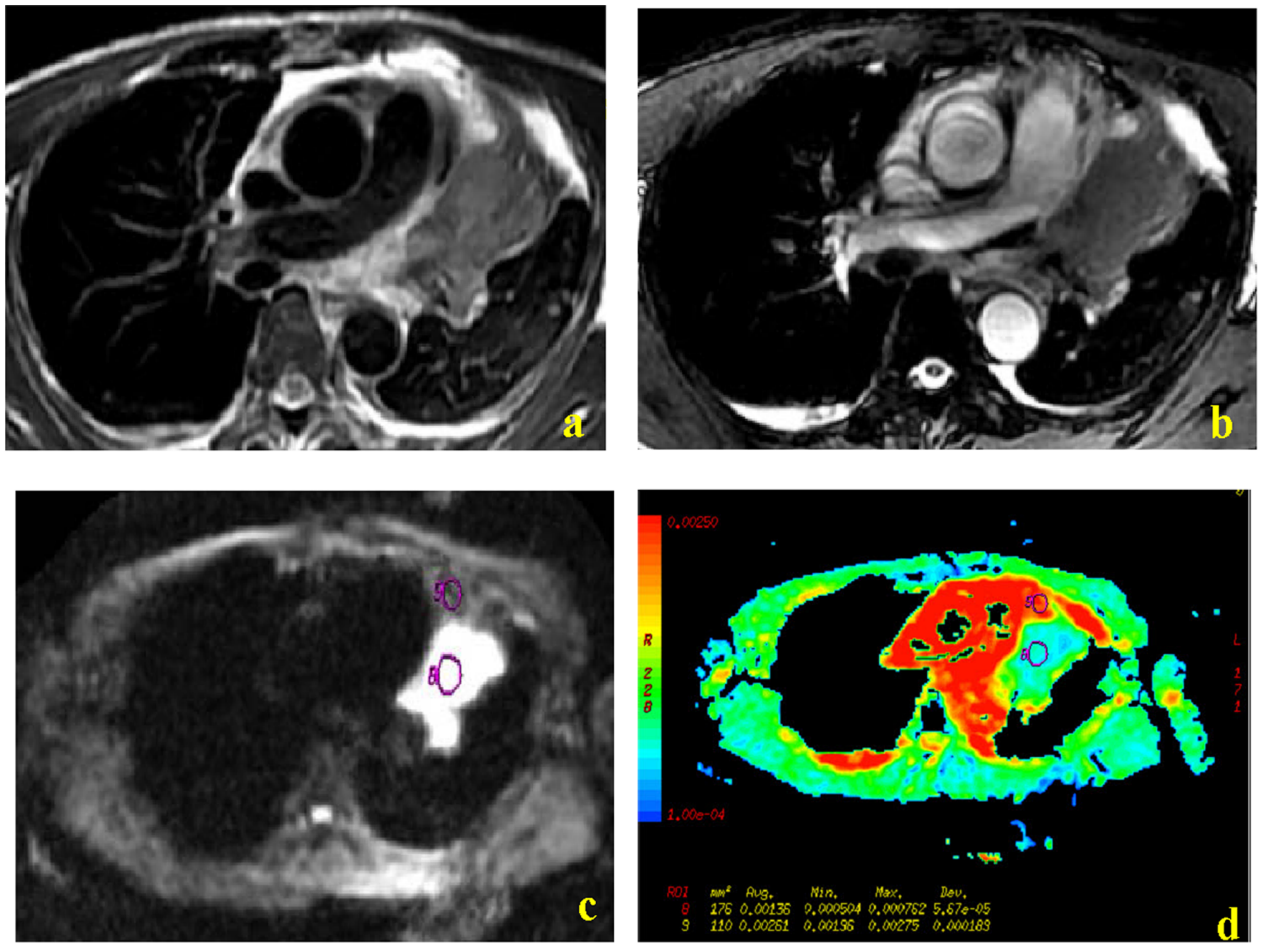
MR images of a 48-year-old man with squamous cell lung carcinoma. FRFSE T2W images and FIESTA images indicate clear hyperintensity of the tumor relative to the atelectasis (a and b, respectively). DW images obtained with a diffusion gradient of 500 s/mm^2^ allowed easy differentiation of tumor and atelectasis (c and d).
